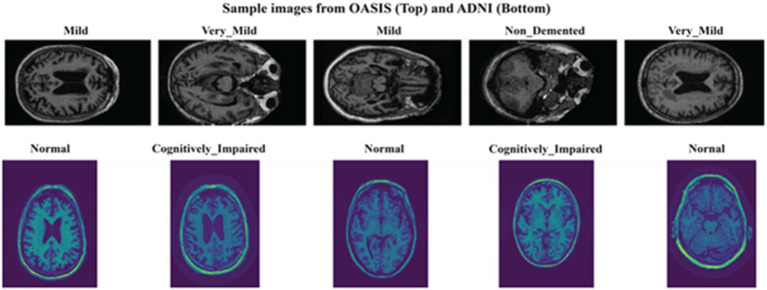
Sample MRI images from OASIS (top row) and ADNI (bottom row) datasets showing different dementia stages.
